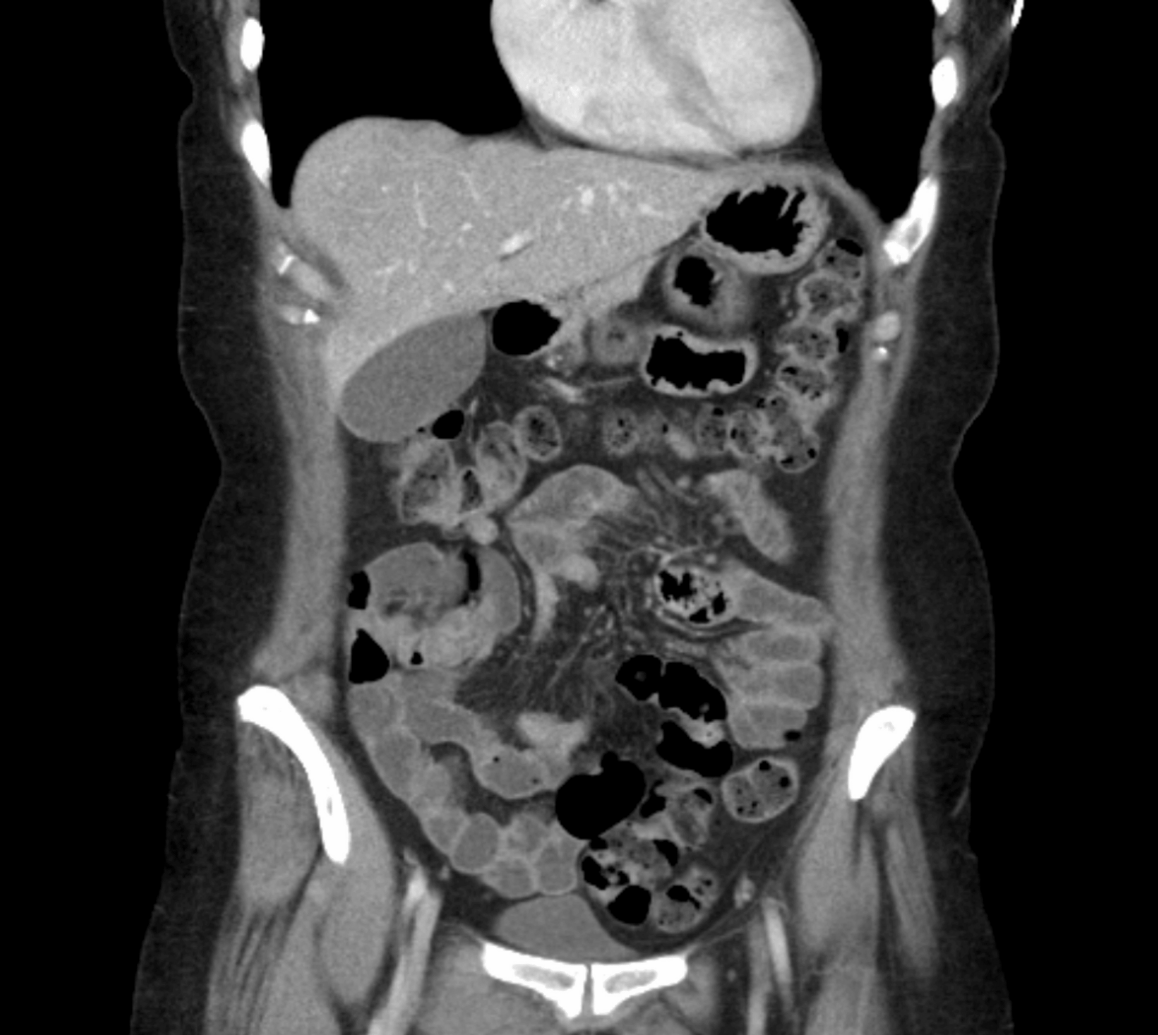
Coronal view of contrast-enhanced CT abdomen pelvis with gallbladder shown
